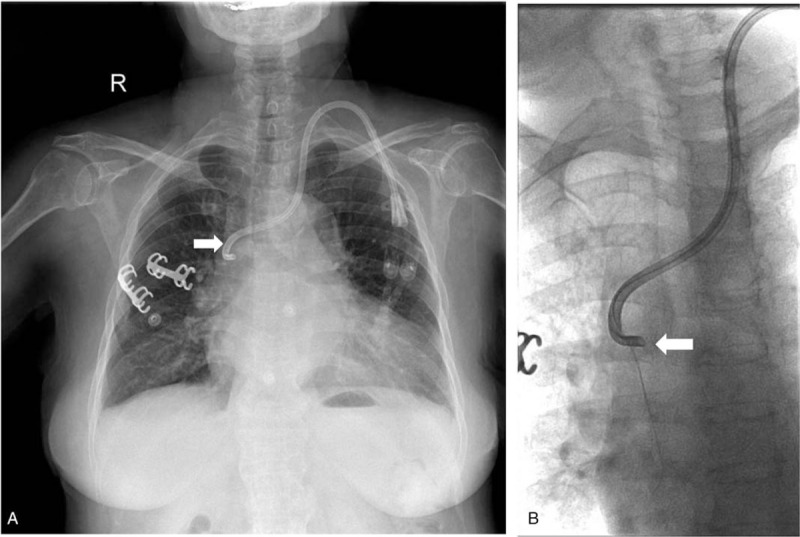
Analysis of catheter tip malposition. (A) Chest radiograph showing catheter tip malposition close to the right tracheobronchial angle structure (azygos vein) like a fishhook (arrow). (B) By injecting contrast into the venous port, DSA confirms that the tip of the catheter is placed in the azygos vein (arrow). DSA = digital subtraction angiography.
